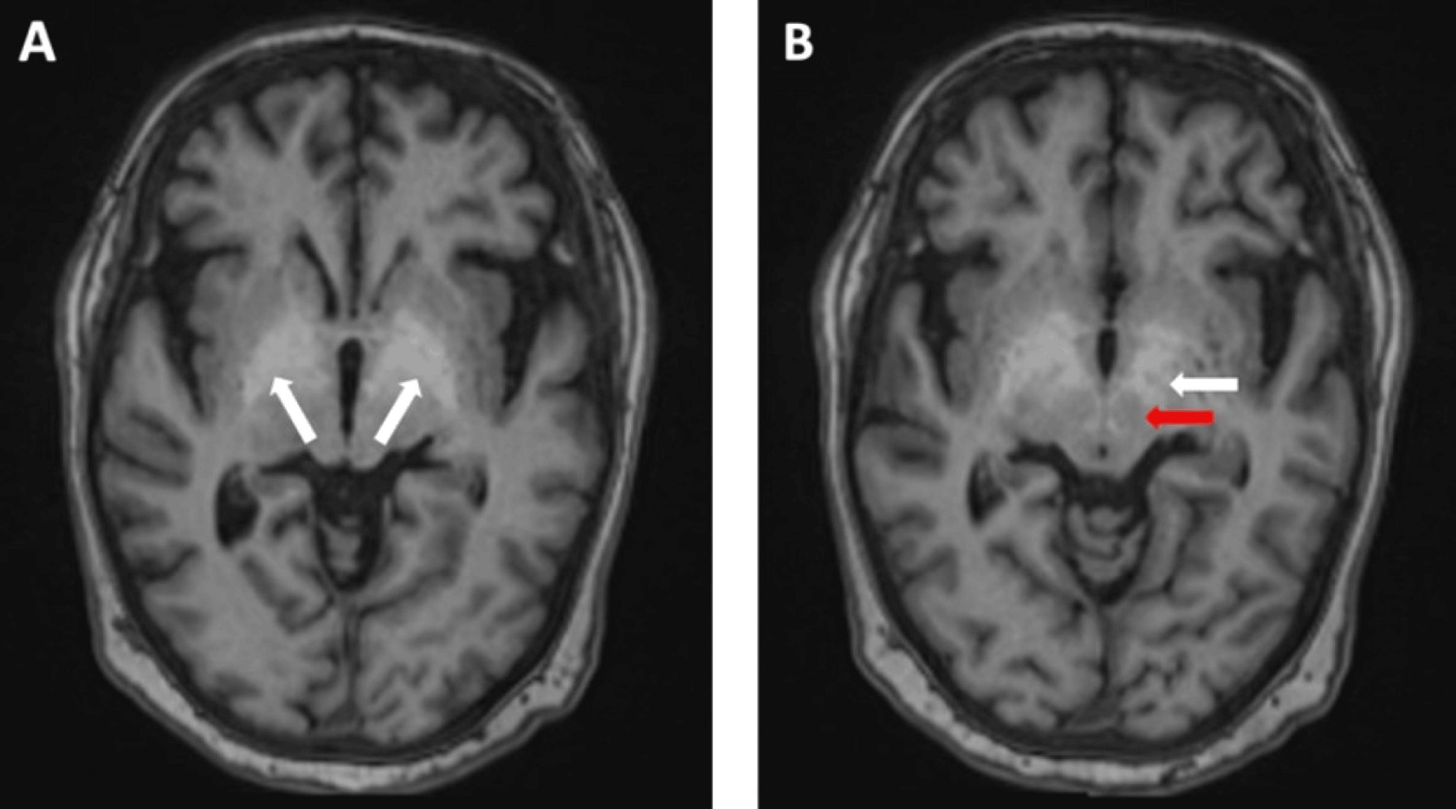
Axial T1-weighted magnetic resonance imaging (MRI) head demonstrating T1 hyperintensity in the globi pallidi (white arrows) in A and the red nucleus (red arrow) and subthalamic nucleus (white arrow) in B, characteristic of manganese (Mn) brain accumulation.
